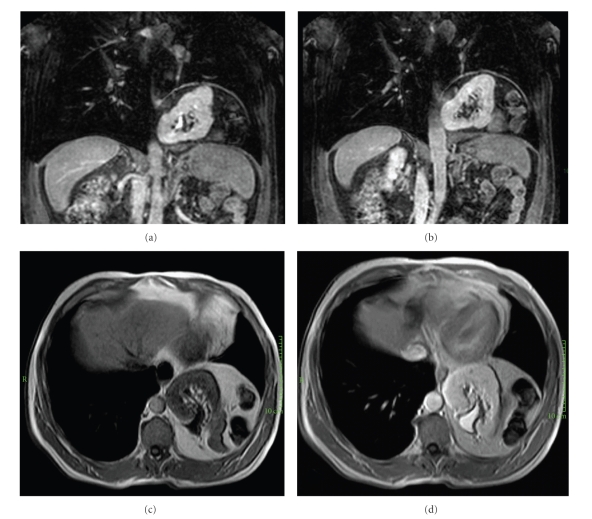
Magnetic resonance imaging (MRI). (a and b) THRIVE sequences with bolus of contrast medium injection; (c) axial T1-weighted sequence; (d) T1 post-Gd DTPA.
